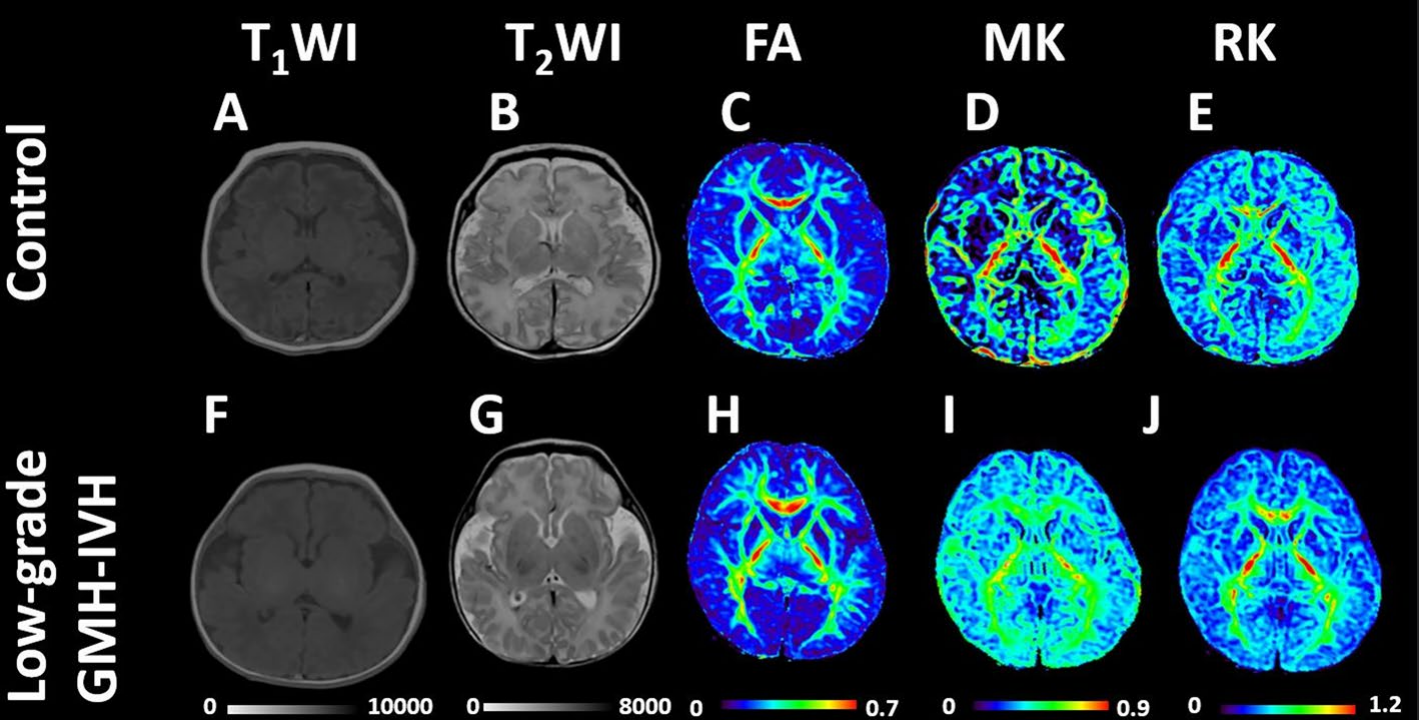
Representative neonatal images (T_1_WI, T_2_WI, FA, MK, and RK) without (top row) and with (bottom row) low-grade GMH-IVH. (A-E) showcases a male neonate with a gestational age of 31 weeks, corrected gestational age at MRI scan is 38 weeks, with Bayley—II scores of MDI and PDI at 125 and 121. (F-J) features a male neonate with a gestational age of 30 weeks, corrected gestational age at MRI scan is 38 weeks, and Bayley—II scores of MDI and PDI at 93 and 100. *DKI* Diffusion kurtosis imaging, *FA* Fractional anisotropy, *MK* mean kurtosis, *RK* radial kurtosis, *low-grade GMH-IVH* low-grade Germinal Matrix Hemorrhage-Intraventricular Hemorrhage, *Bayley—II* Bayley Scales of Infant and Toddler Development 2nd Edition, *MDI* Mental Development Index, *PDI* Psychomotor Development Index.
